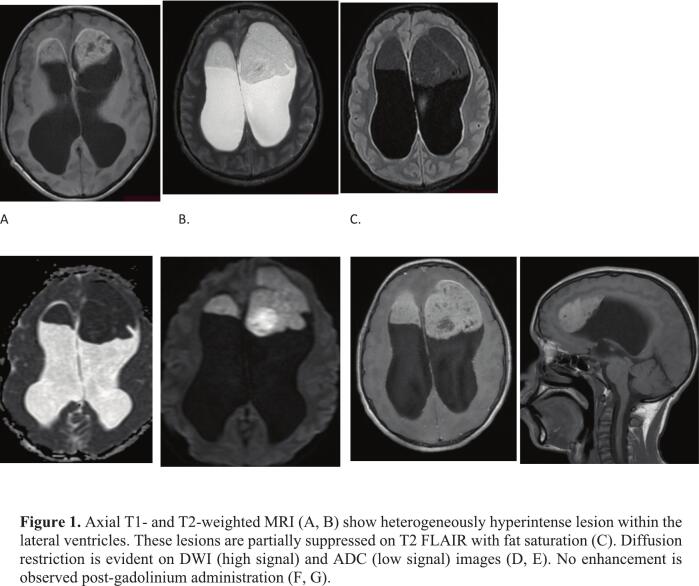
Axial T1- and T2-weighted MRI (A, B) show heterogeneously hyperintense lesion within the lateral ventricles. These lesions are partially suppressed on T2 FLAIR with fat saturation (C). Diffusion restriction is evident on DWI (high signal) and ADC (low signal) images (D, E). No enhancement is observed post‑gadolinium administration (F, G).
